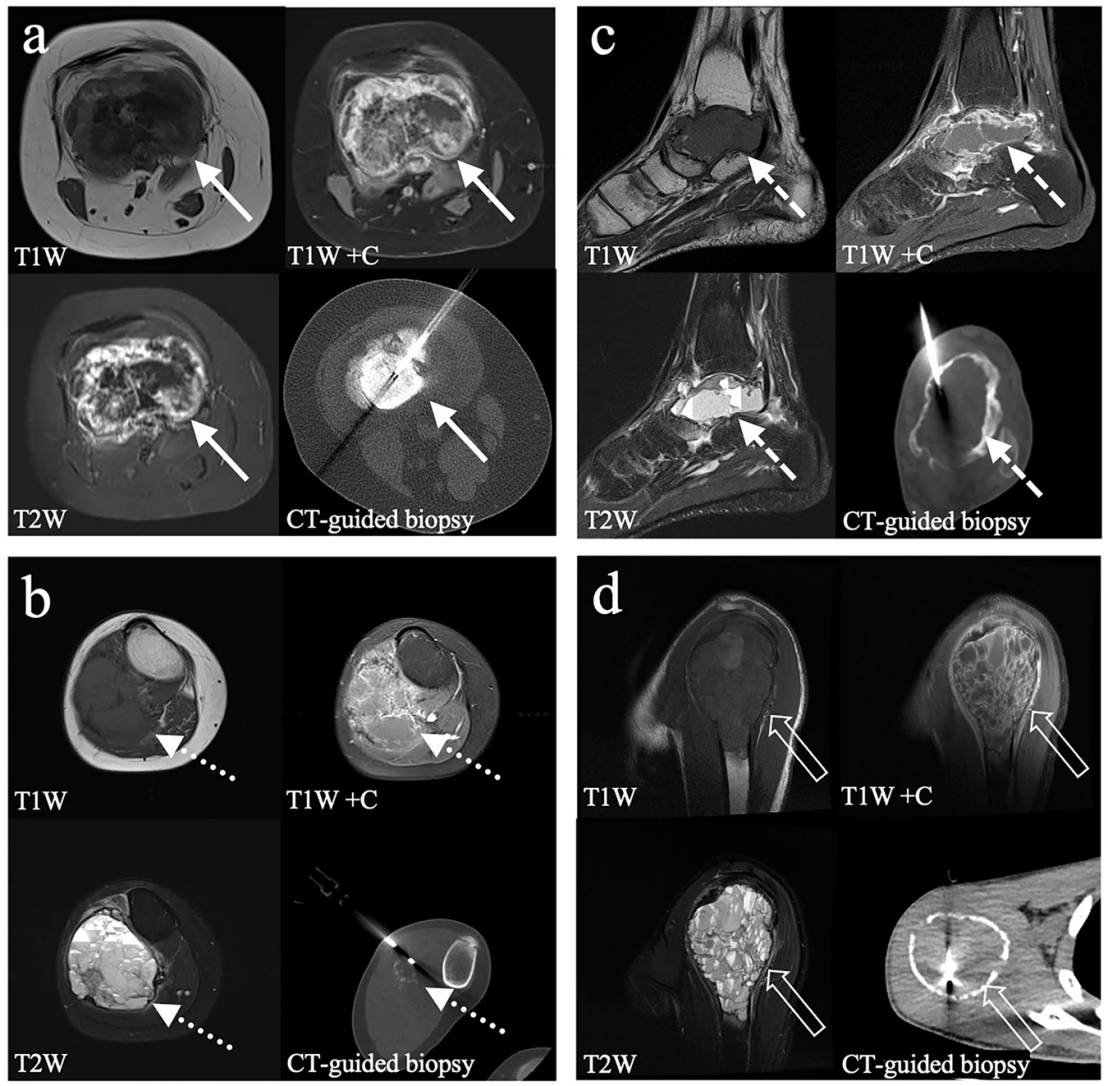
Bone lesions with pre-biopsy contrast-enhanced MRI were categorized according to their proportion of solid and cystic content. a Solid lesion (> 90% solid component) distal femur—final diagnosis: osteosarcoma (solid arrows). b Predominantly solid lesion (50–90% solid component) proximal fibula—final diagnosis: ewing sarcoma (dotted arrows). c Predominantly cystic lesion (> 50–90% cystic component) talus—final diagnosis: chondroblastoma (dashed arrows). d Cystic lesion (> 90% cystic component) proximal humerus—final diagnosis: aneurysmal bone cyst (void arrows)
